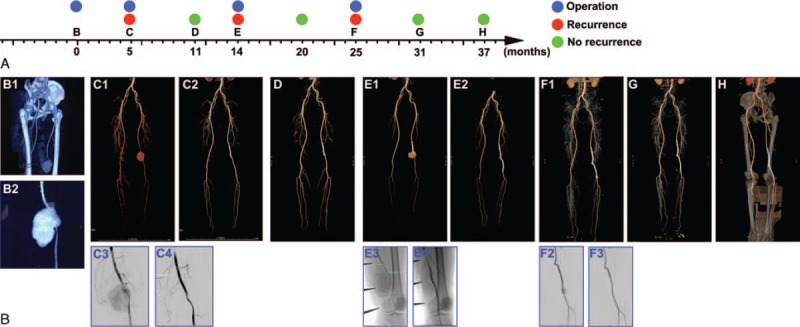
Endovascular treatment of BD with recurrent infrainguinal arterial pseudoaneurysms: a case report and literature review. (A) A sketched map with a time axis showing the therapeutic treatments and follow-up examinations of the reported patient with v-BD. Colored pie charts represent specific events (surgery, recurrence, or no recurrence) at each time point. (B–I) CT angiography of the infrainguinal arteries or arteriogram of the reported patient with v-BD at time points as indicated in (A). For Figure 1C, Figures 1C1 and 2 are the CT angiograms of this patient obtained before and after endovascular treatment, respectively. Figures 1C3 and 4 are the arteriograms obtained before (C3) and immediately after (C4) the implantation of the covered stent graft during the endovascular treatment of the aneurysm. Figures 1E and G, Figures 1E2, G2, E3, and G3 show the arteriograms obtained before (E2, G2) and immediately after (E3, G3) the implantation of the covered stent-graft during the endovascular treatment of the aneurysm. BD = Behcet disease, CT = computed tomographic, v-BD = vasculo-Behcet disease.
